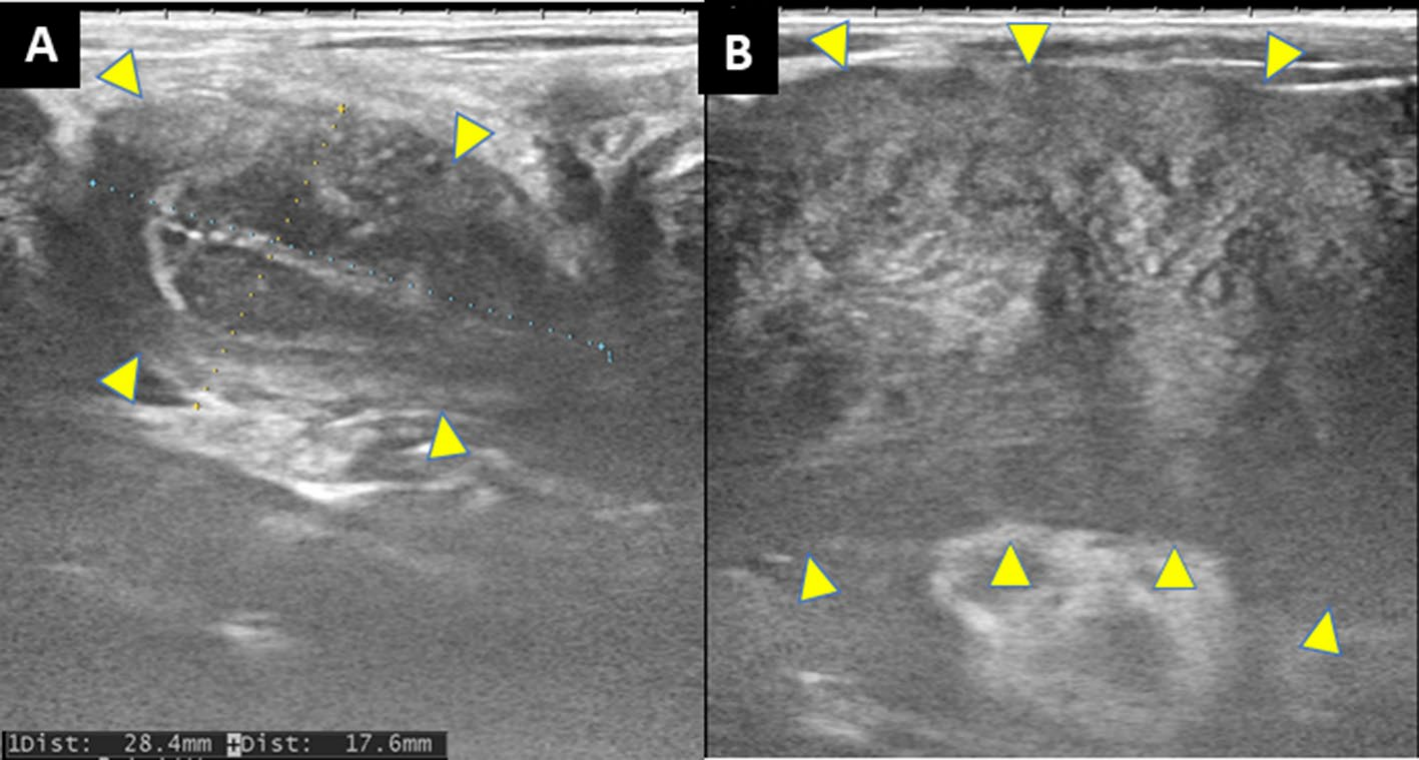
Transabdominal ultrasonography of the long axis of the uterine body in case 5. (A) The uterine body (28.4 × 17.6 mm: long axis × anteroposterior diameter) after surgery. (B) An enlarged uterine body (60.0 × 28.1 mm: long axis × anteroposterior diameter) without an endometrium was found at the time of diagnosis of irreversible rejection.
